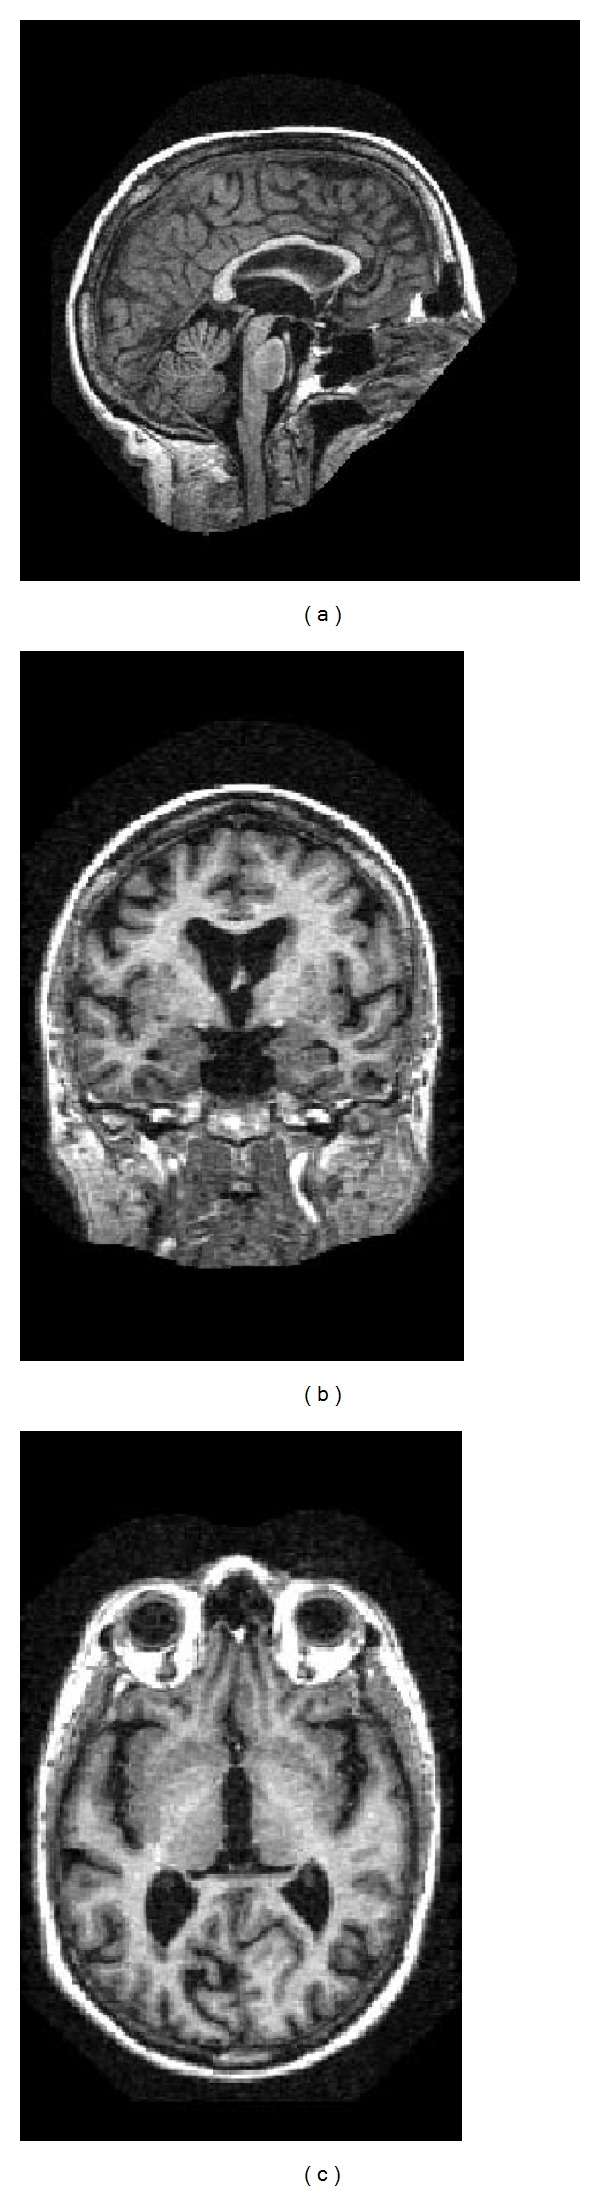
The three projections of the MR image in Figure 1: (a) sagittal, (b) coronal, and (c) axial.
